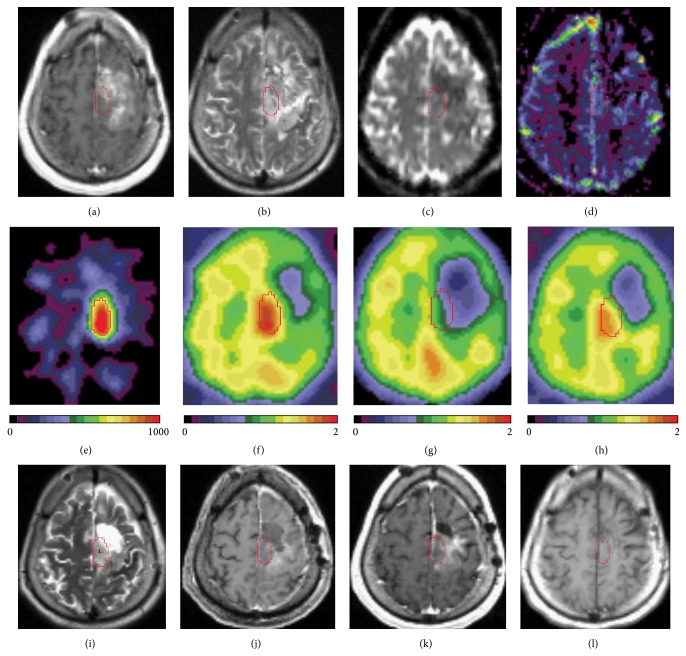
Coregistered PET and MR images from individual with anaplastic oligodendroglioma WHO grade III (participant #14). ((a)–(d)) MR images obtained at the time of PET study. (a) T1-weighted MR image with contrast (Gadolinium) enhancement; (b) T2-weighted MR image; (c) Apparent Diffusion Coefficient (ADC) diffusion-weighted MR image; (d) Cerebral Blood Volume (CBV) perfusion-weighted MR image; (e) AG PET image; (f) CMRGlu PET image; (g) CMRO_2_ PET image. (h) CBF PET image; (i) T2-weighted MR image 1 day after surgery (2 days after the PET study); ((j)–(l)) Follow-up T1-weighted images with contrast (Gadolinium) enhancement obtained 2 days (j), two months (k), and 14 months (l) after the PET study.
